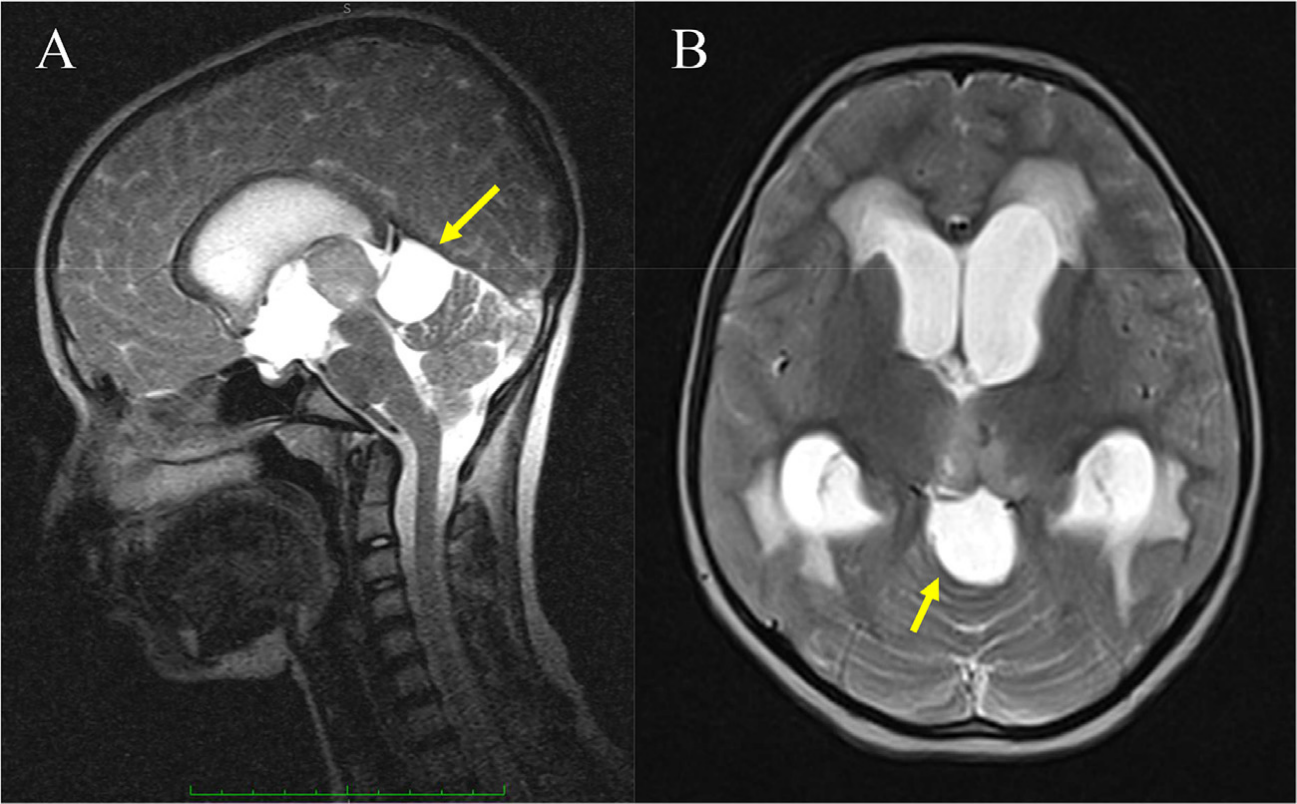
Sagittal (A) and Axial (B) T2W MRI image demonstrated enlargement of the lateral and third ventricular system secondary to quadrigeminal cistern arachnoid cyst compressing the brainstem, cerebellum and aqueduct of sylvius is obstructed and occupying quadrigeminal plate cistern causing hydrocephalus.
